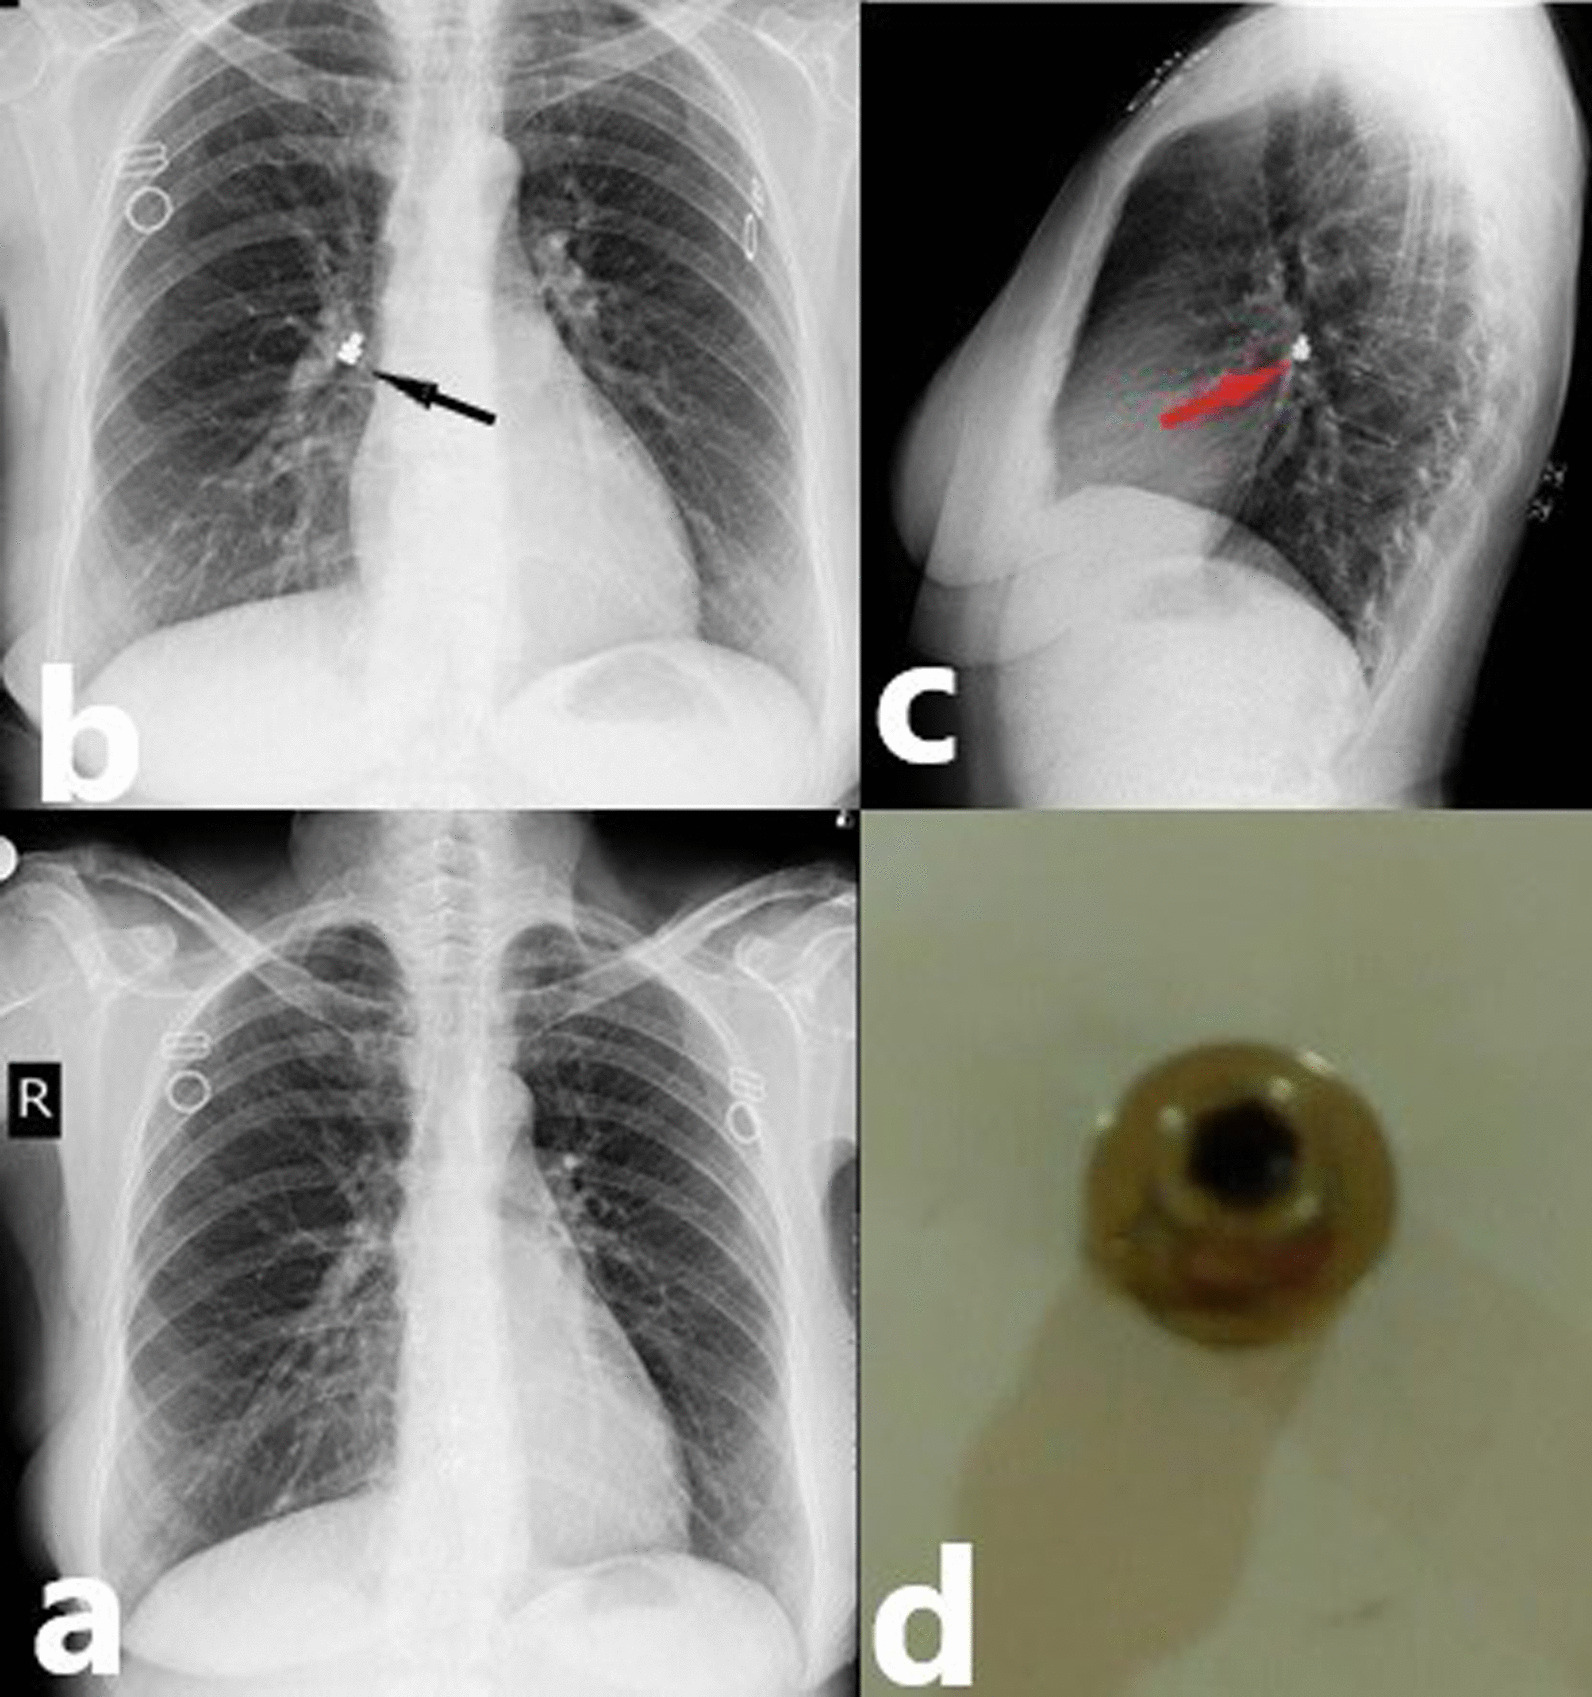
Dental screw expectorated by cough. a Posterior-Anterior (PA) chest X-ray; after expectorated. b, c Posterior-Anterior (PA) and lateral chest X-ray; black and red arrow shows dental screw. d Dental screw
